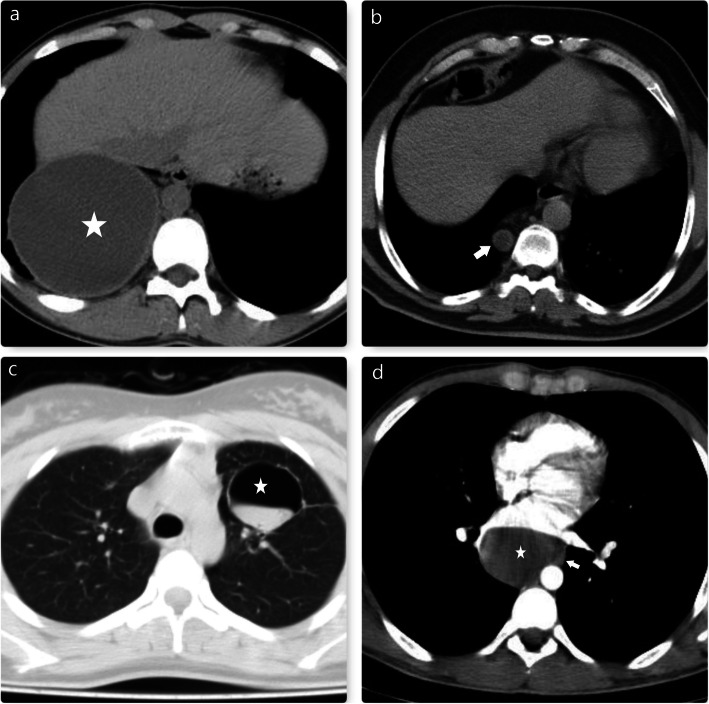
a. Chest CT indicates the right-sided giant enteric cyst (Asterisk). In this case, we preferred open surgery to performed complete resection. b. A much smaller enteric cyst is observed than in the previous case (Arrow). Therefore, we have preferred VATS for the excision of this cystic lesion. c. Thorax CT image of the left-sided congenital cystic adenomatoid malformation is seen (Asterisk). The left upper lobectomy was performed. d. This thorax CT belongs to the patient with an esophagus duplication cyst (Asterisk). Arrow indicates the wall of the esophagus. There is no contraindication, but these areas of the mediastinum are challenging localizations for the VATS procedure. Thus, we preferred open surgery in this case
